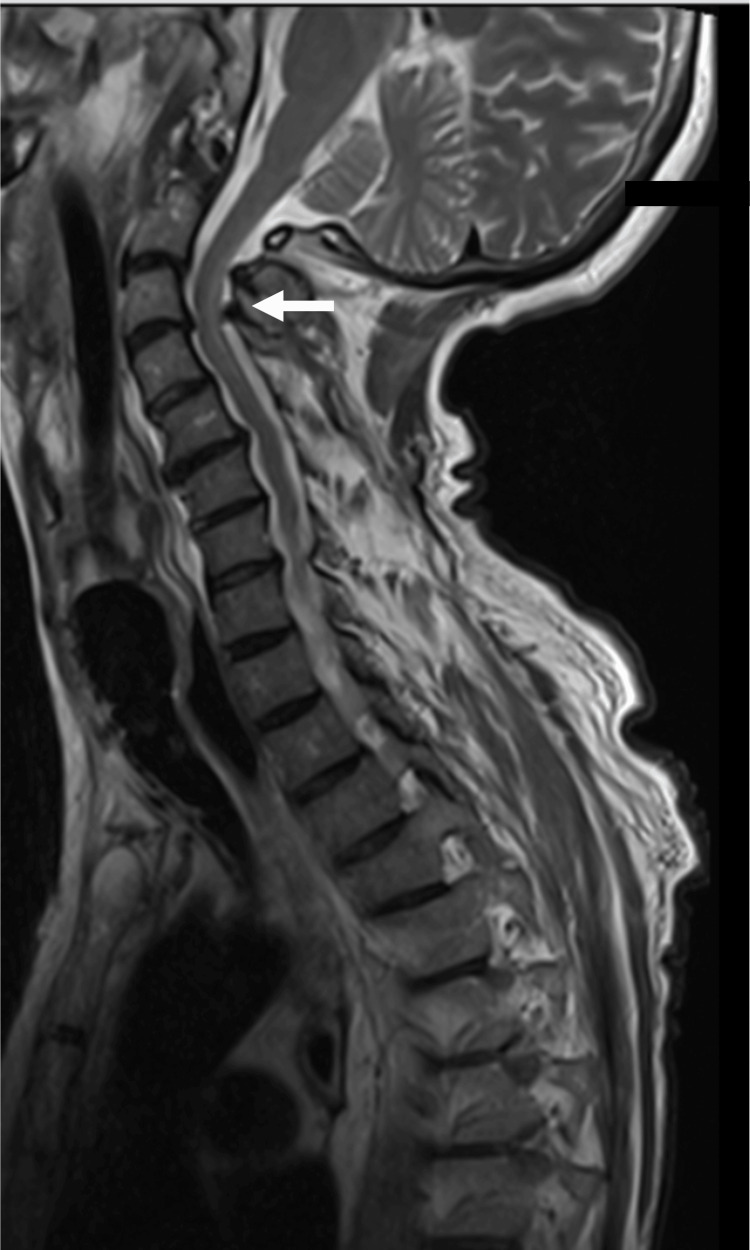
Sagittal T2 MRI of the cervical spine showing T2 hyperintensity in the dorsal columns
